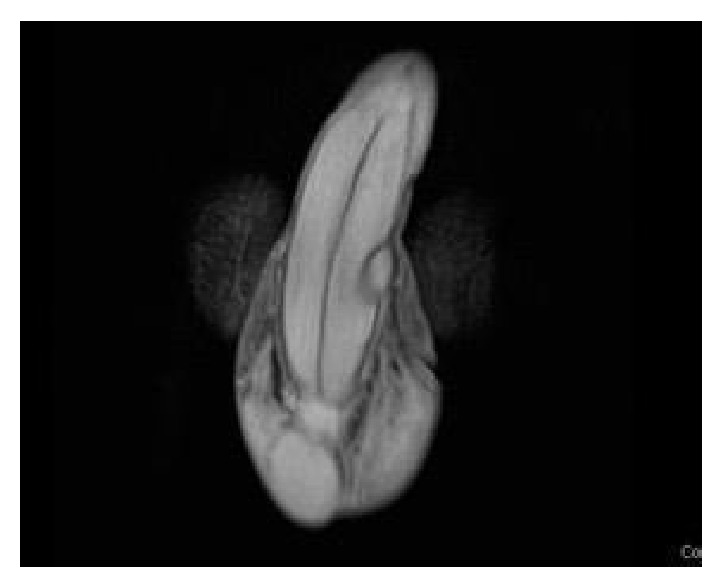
MRI of the penis T2 coronal. Dorsolaterally located nodule: clearly separate from tunica albuginea but included in Buck's fascia.
